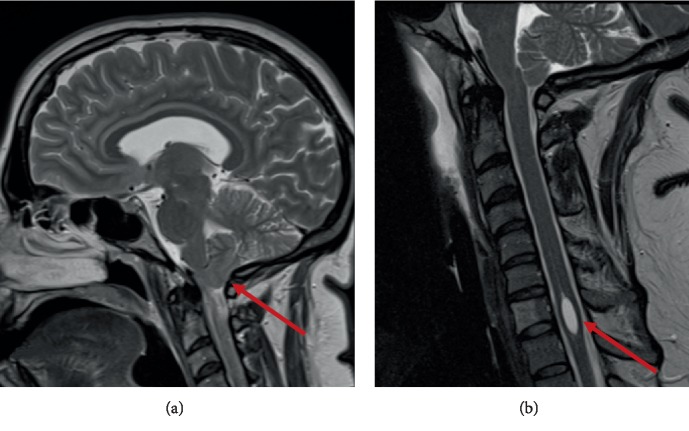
T2-weighted MRI demonstrating Arnold-Chiari I malformation. (a) shows a 7 mm downward displacement of the right cerebellar tonsil (red arrow). (b) shows a syrinx centered about C7-T1 measuring 17 mm and 5.6 mm in the largest longitudinal and axial dimension (red arrow). A benign hemangioma of C7 anterior vertebral body is also identified.
